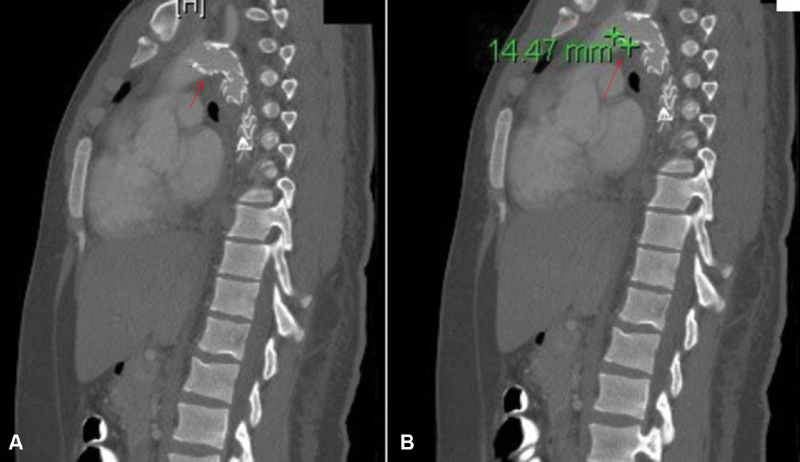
Sagital view for chest computed tomography shows inner (medial) lip for the stent graft that increased the risk for collapse as shown in arrow (
